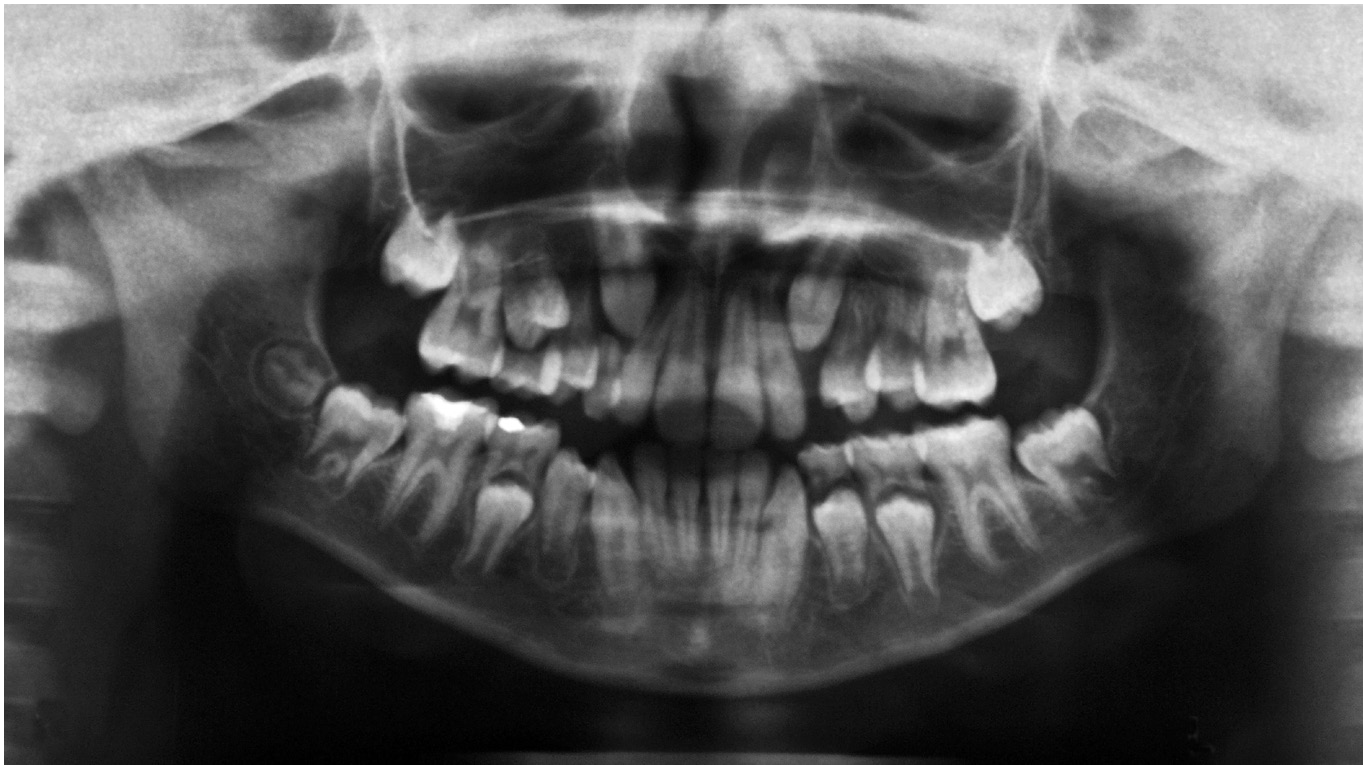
Initial panoramic radiograph.
